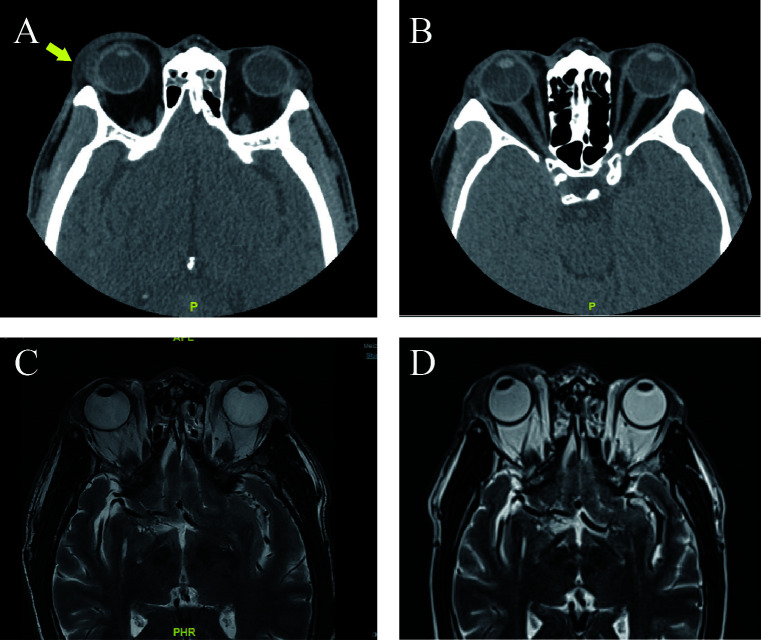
Radiological imaging of the orbits. (A) Preoperative axial computed tomography (CT) scan revealing soft tissue swelling and a small nodule (arrow) in the medial aspect of the right upper eyelid. (B) Postoperative axial CT scan at 3 days confirming successful removal of the right orbital mass. (C) One-month postoperative coronal T2-weighted magnetic resonance imaging (MRI) showing bilateral lacrimal gland and surrounding soft tissue swelling. (D) Postoperative coronal T2-weighted MRI at 2 years showing no evidence of disease progression.
